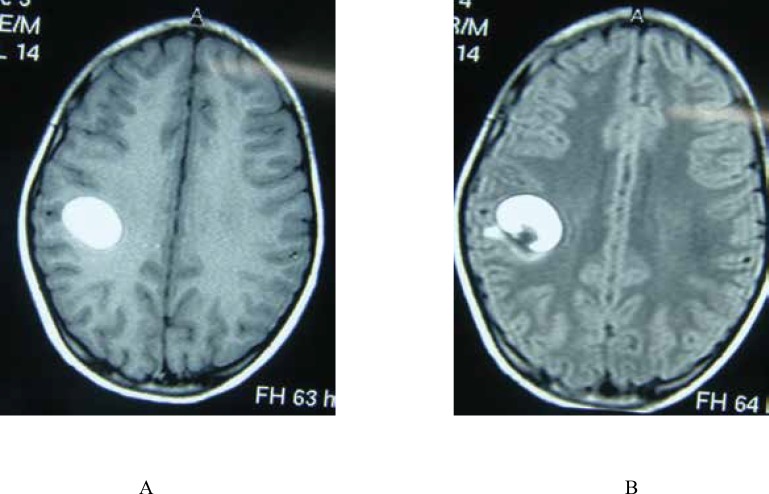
An 11-Year-Old Boy With FUS : T1W (A) and FLAIR (B) images reveal high signal intensity lesion in the left parietal lobe with hyposignal internal component due to hematoma
